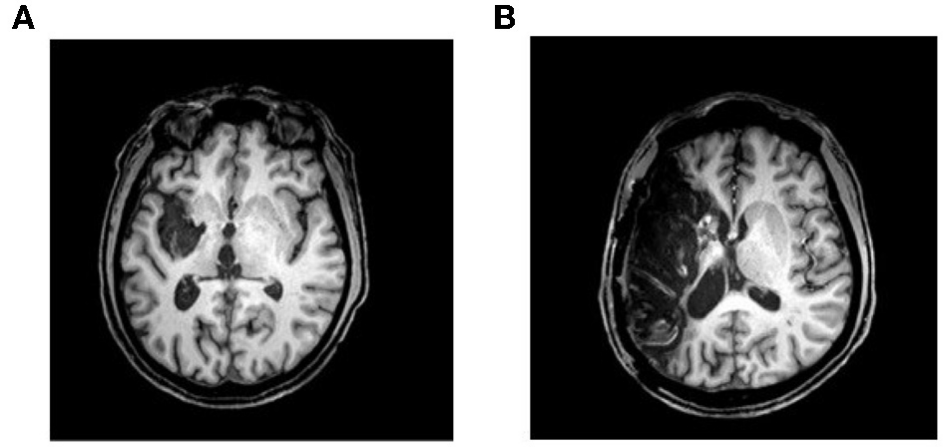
Structural images of the left hemisphere lesions for participants (A) GOE and (B) AMC. Participant AMC had a titanium mesh plate inserted into his left temporalis muscle following his stroke. The metal inserted into AMC's skull interfered with the MRI scan of that left region and, therefore, it is difficult to pinpoint the exact location of his lesion on the image.
